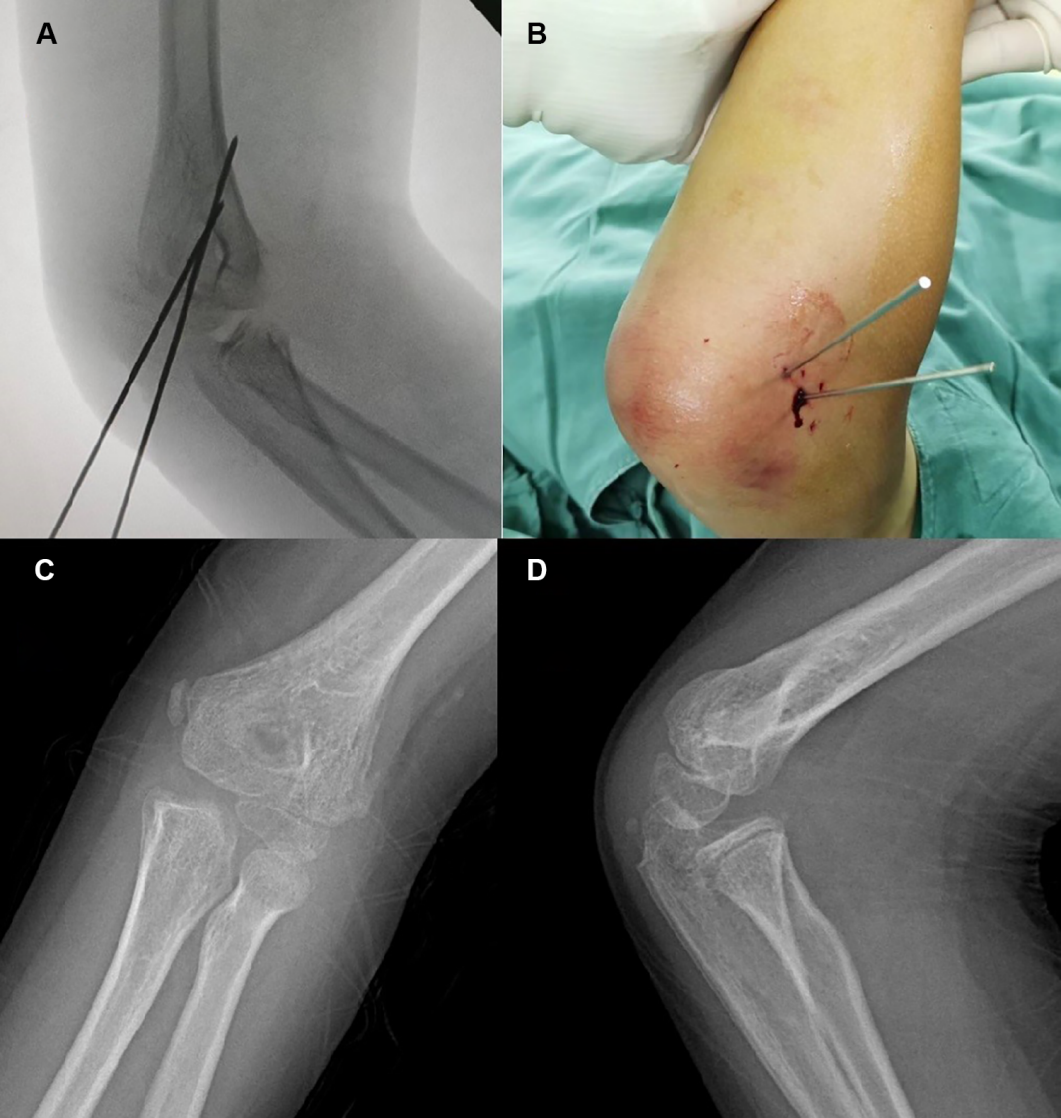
Typical x-ray of Type III fractures. Internal oblique (A) image of the fracture showed satisfactory reduction. Aspect (B) of the elbow after K-wire fixation. AP (C) and lateral (D) radiographs 5 months postoperatively.
